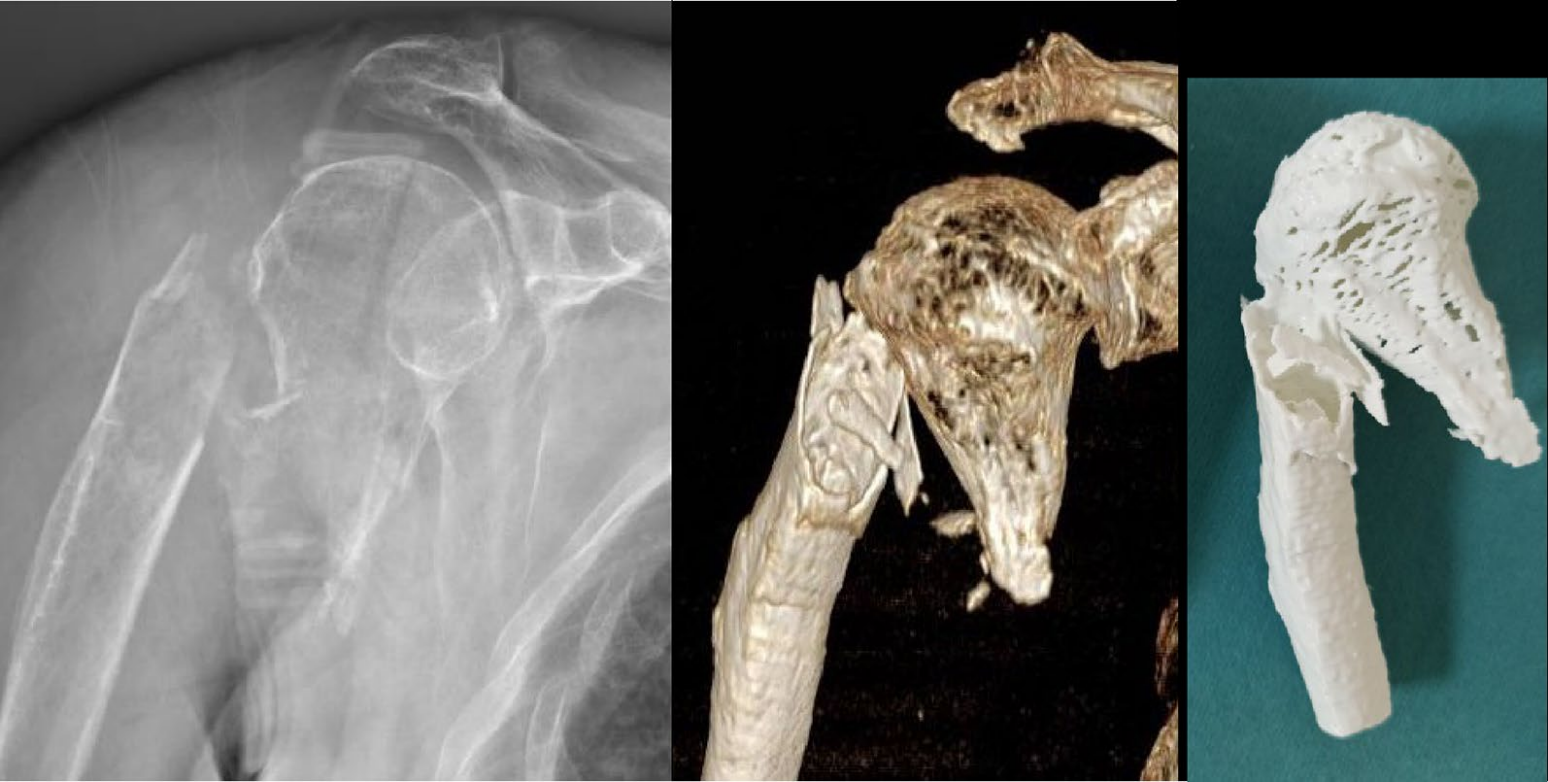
From radiography to CT with 3D volumetric rendering to full-size 3D-printed fracture replica: the transformation of surgical planning from the virtual stage to the real stage
